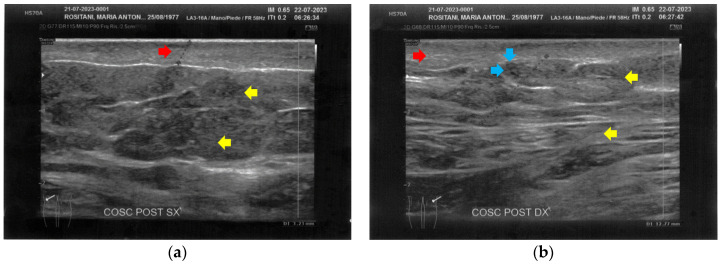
Ultrasonography of the posterior thighs at T1. (a) On the left side, an improvement of the cicatricial fibrosis (red arrow) is clear. A restructuring of all the layers (yellow arrows) is also present; (b) The right side presents the same improvements as the left. The appearance of superficial vascular elements (blue arrows) is evident.
